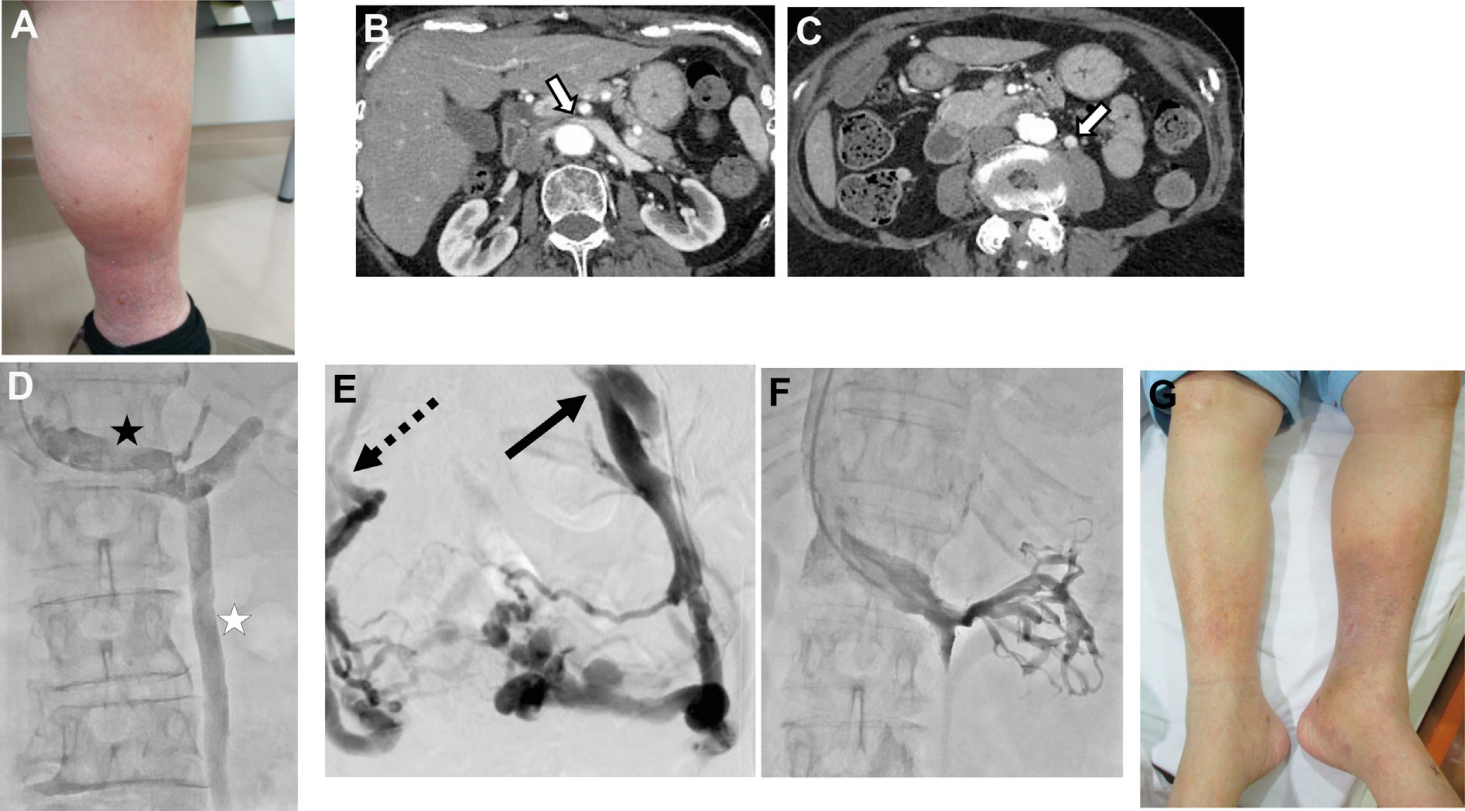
Case 1. (A) Preoperative photograph of the patient demonstrating swelling, hyperpigmentation, and serous exudate on her left lower extremity. (B) Computed tomography angiography (CTA) showing the left renal vein (LRV) compression between the superior mesenteric artery and the aorta (white arrow). (C) Early opacification of the left gonadal vein (LGV) (white arrow). (D) Venogram shows compression of the LRV (black star) and the collateral pathway (LGV reflux) (white star). (E) Selective left gonadal venography demonstrates LGV reflux drained into the left common iliac vein (CIV) (black arrow) via the pelvic venous plexus (some into the right internal iliac vein; dashed black arrow). (F) After LRV stenting, the collateral pathway was reduced. (G) The symptoms in her left lower extremity improved postintervention day 1.
